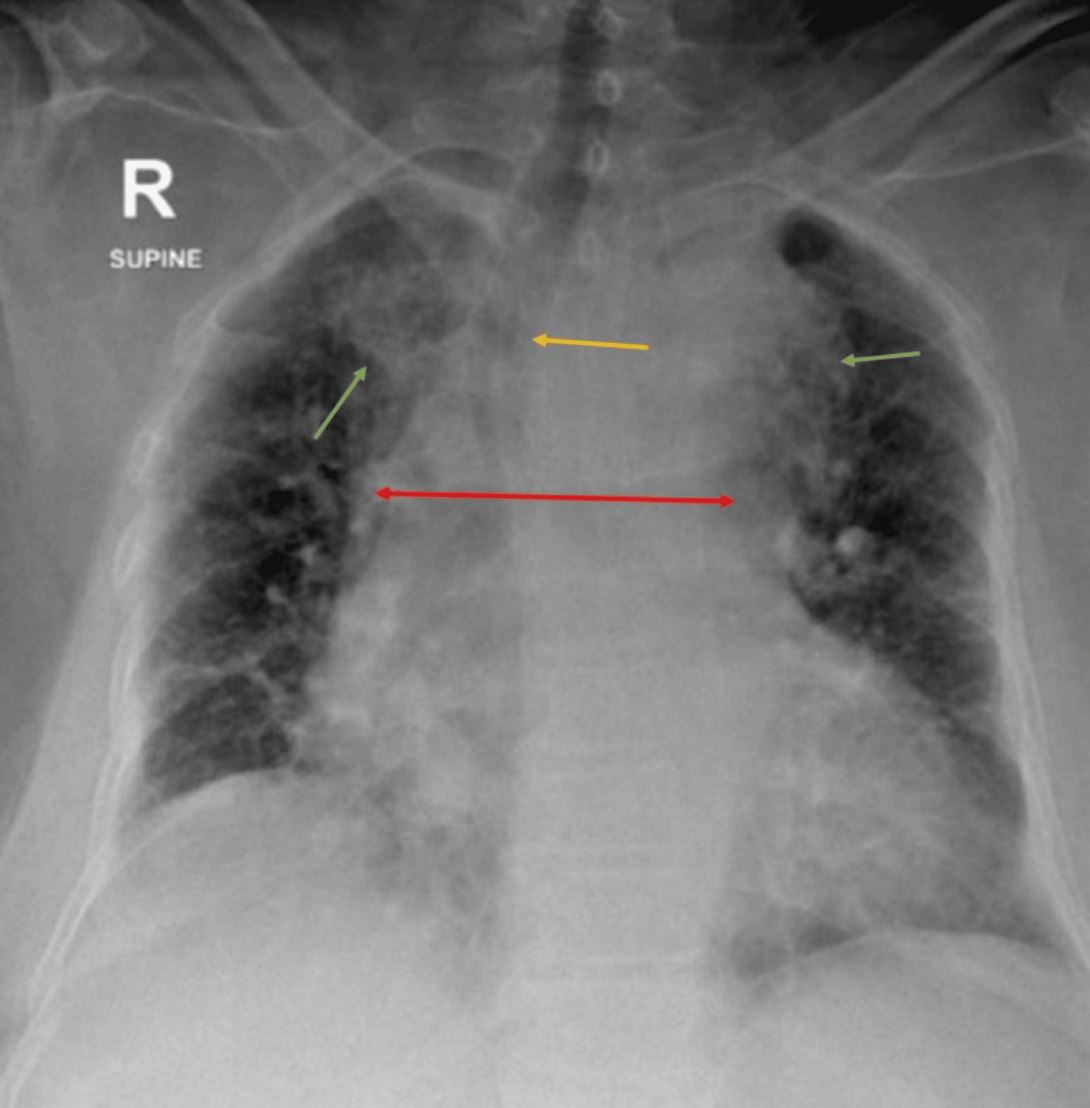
Chest X-ray taken on day 1 post-admission, showing bilateral upper lobe consolidation (green arrows), mediastinal widening (red arrow), and a trachea shifted to the right side (yellow arrow)
